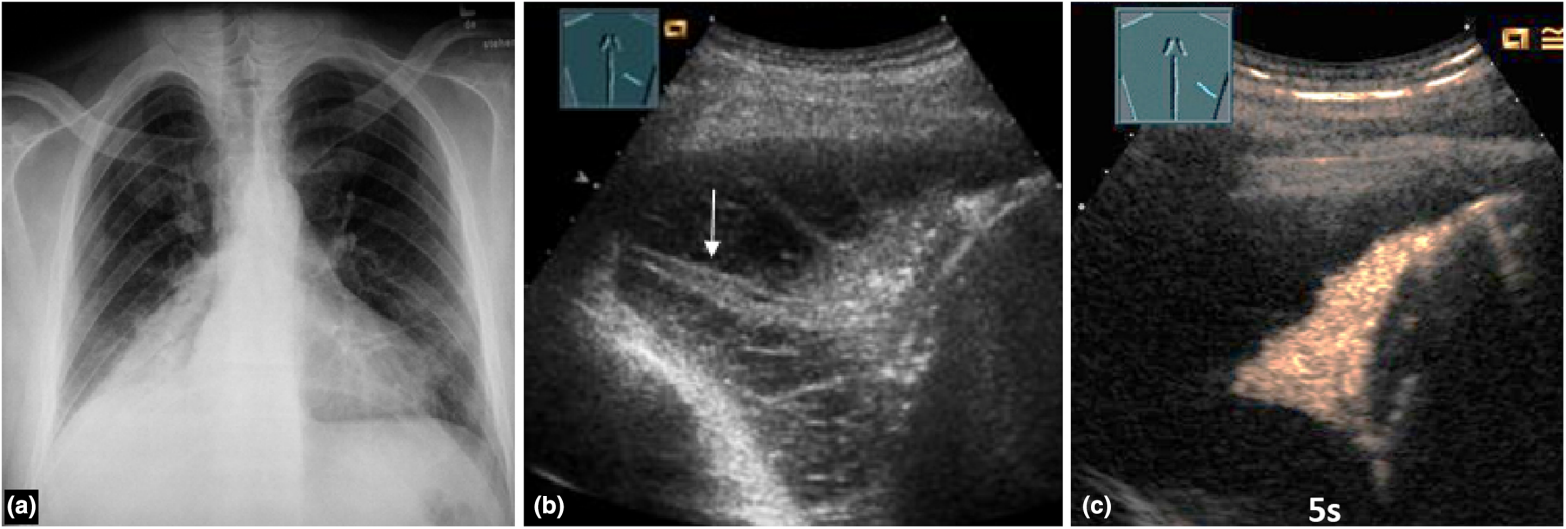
A 66‐year‐old patient presented to the emergency department with severe dyspnea, right thoracic pain, severe cough, and yellowish sputum with hemoptysis, and with subfebrile temperatures. Visualisation of a right‐sided, paracardial consolidation on chest X‐ray (courtesy of Prof. Dr. Andreas H. Mahnken, Department of Radiology, University Hospital Marburg) (a); B‐mode ultrasound (b) reveals a hypoechoic consolidation with central air bronchogram and multiple fibrin septa (arrow); on contrast‐enhanced ultrasound (c), the consolidation after 5 seconds demonstrates a homogeneous, pulmonary arterial pattern of enhancement, where the fibrin threads show an absence of enhancement consistent with pneumonia with parainfectious polyseptated effusion. Thoracoscopic pleural decortication was performed.
